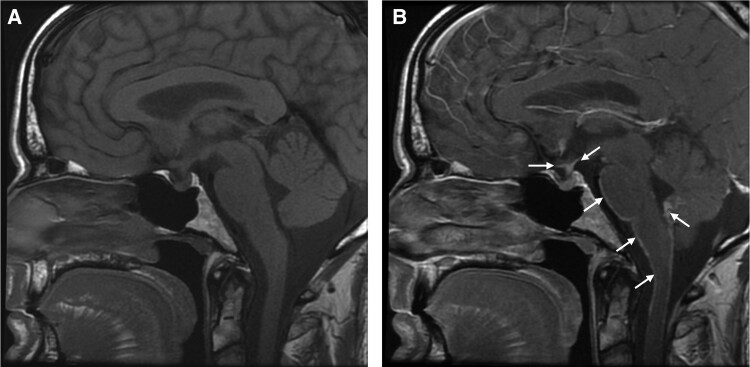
Case 3: T1-weighted MRI pre- (A) and post- (B) gadolinium. The posterior pituitary bright spot is absent on the noncontrast imaging. Following gadolinium administration, there is abnormal enhancement along the floor of the third ventricle (upper two arrows), together with widespread leptomeningeal enhancement (lower four arrows).
